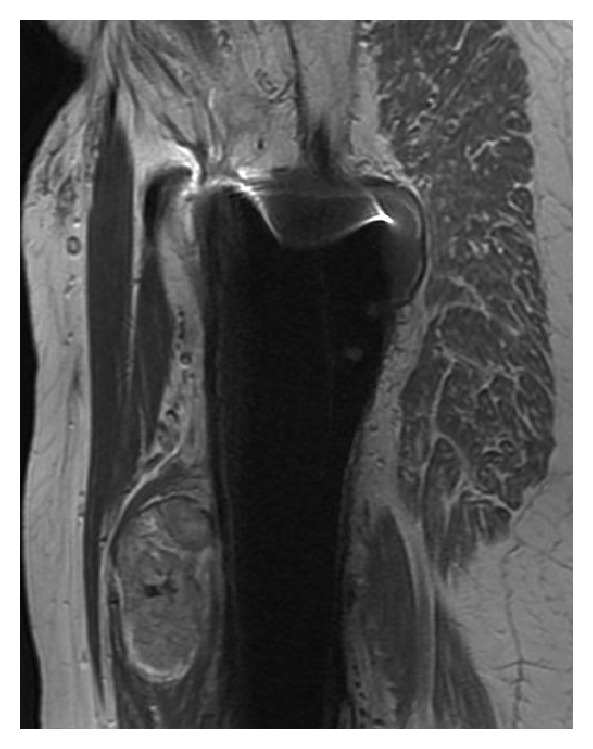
Sagittal T1-weighted MR image. The lesion is well defined at the prosthesis bone junction.
